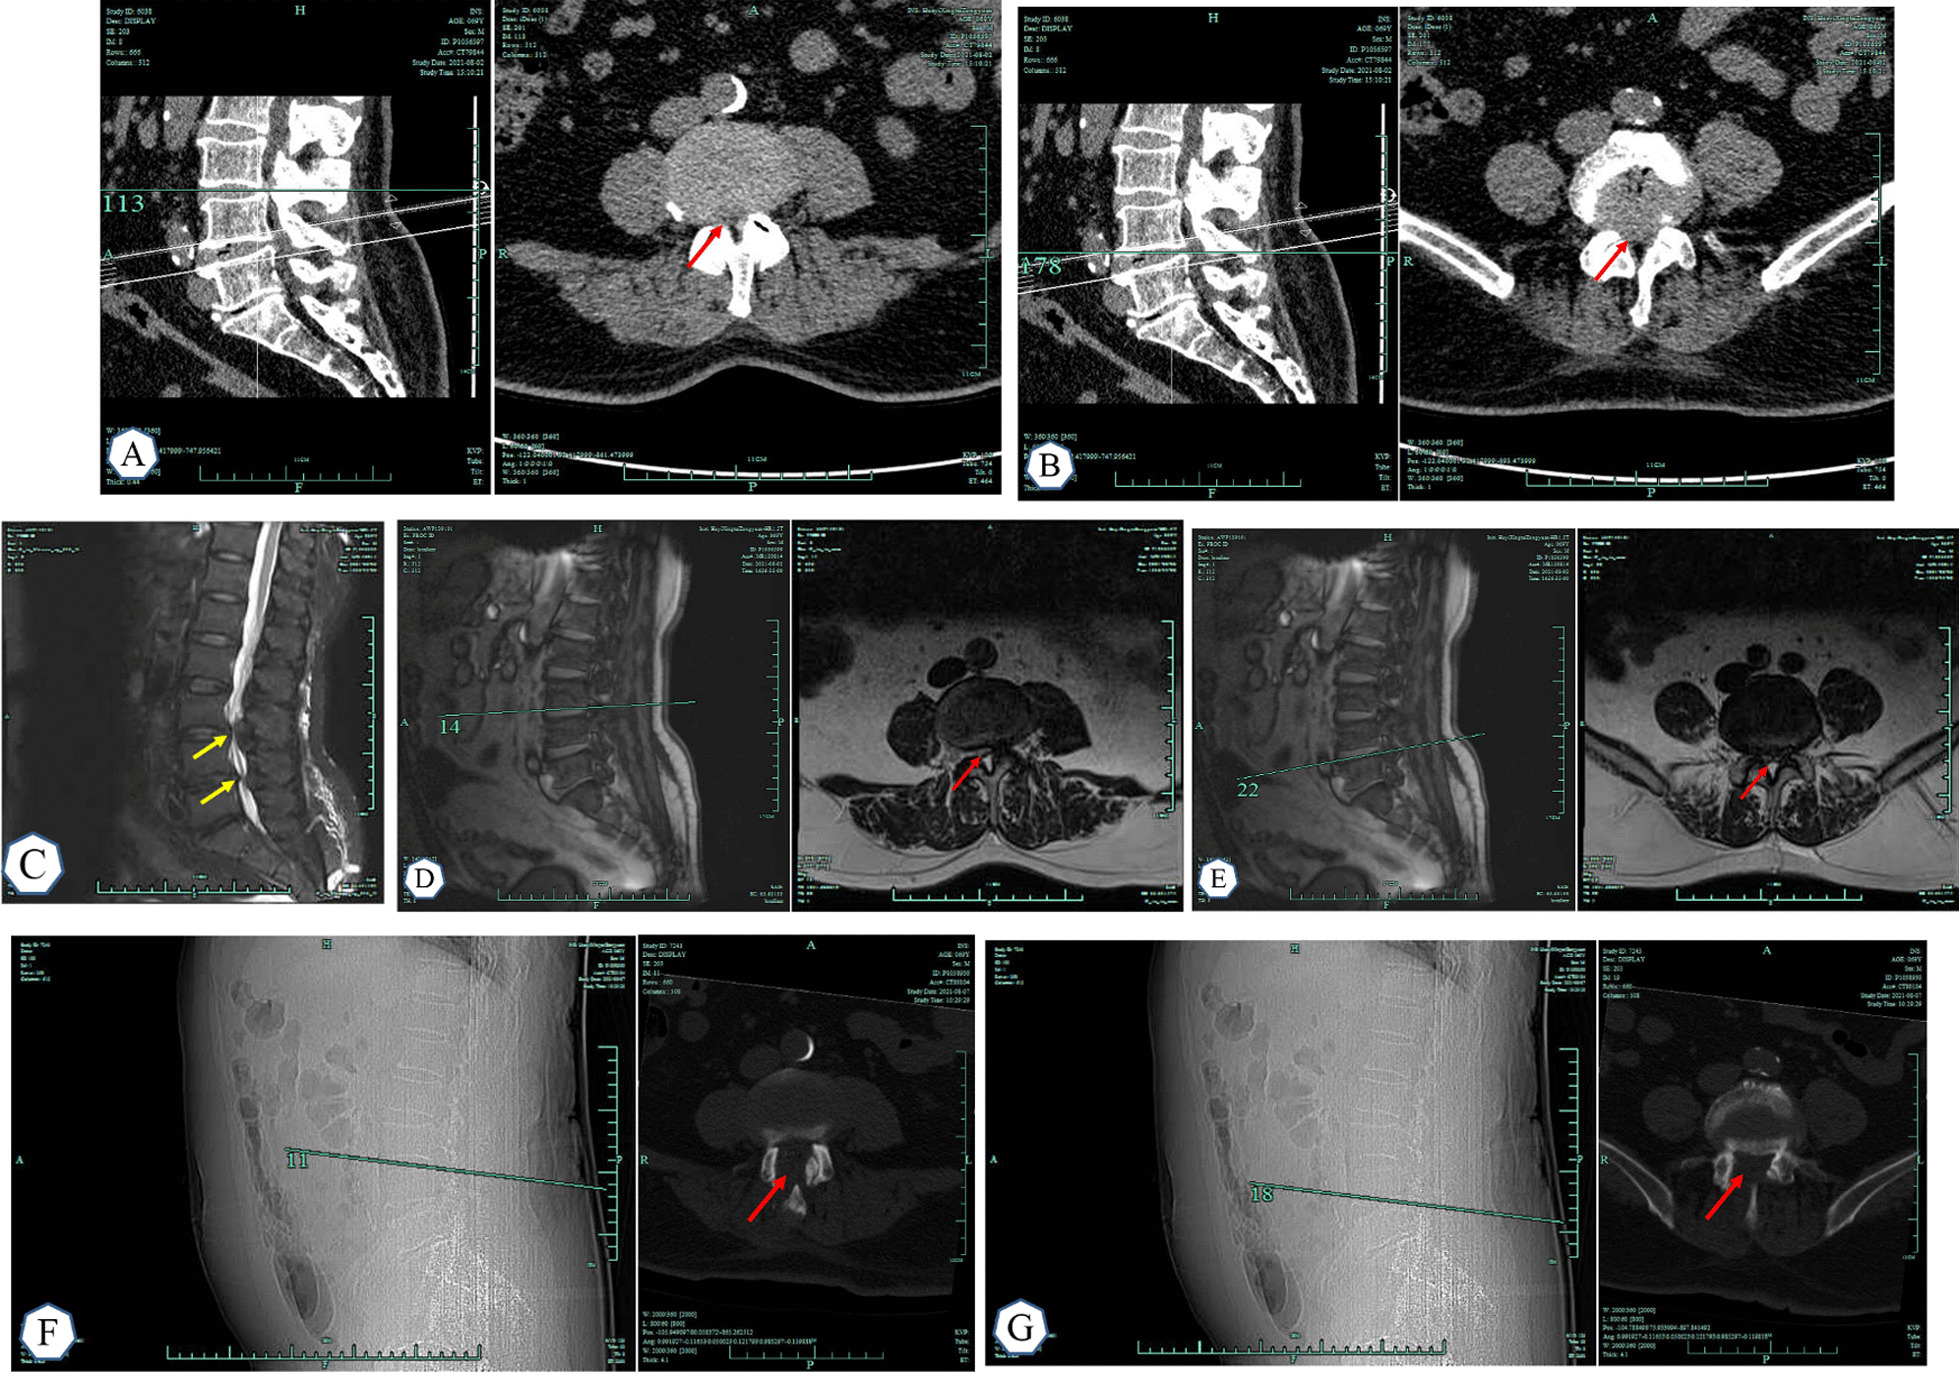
Typical case A–E showed CT and MRI examinations of the lumbar spine before surgery. Arrows showed L3–L5 lumbar spinal stenosis area.The arrows in (F) and (G) show the decompression area during the postoperative CT examination
